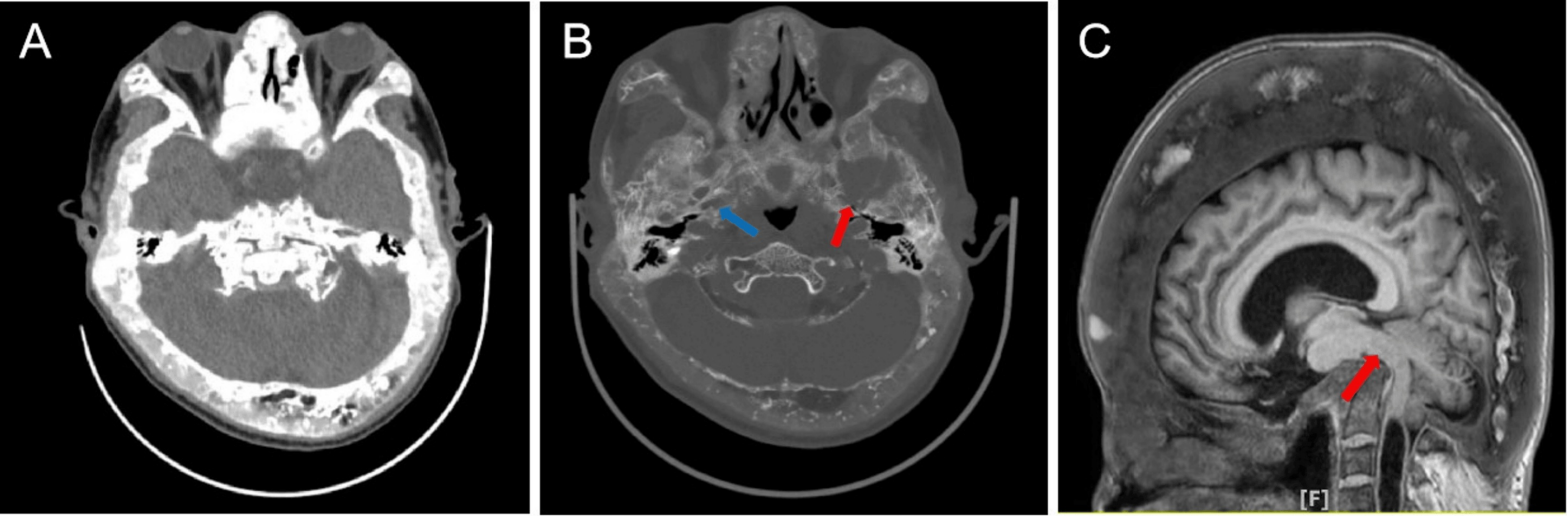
Imaging manifestations (A, B) Preoperative CT of the head. (A) Diffuse thickening of the cranial bones and uneven bone density was seen on 1.25 mm Stnd. (B) The affected foramen ovale (red arrow) appeared less distinct compared to the opposite side(blue arrow) was seen on the 1.25 mm bone. (C) MRI scans showed diffuse thickening of the cranial bones, primarily in the outer plate, with nodular and cord-like mixed signals within the diploic space. Other findings included clear gray-white matter differentiation, slightly elongated T1 and T2 signal bands around the lateral ventricles, normal ventricular and cisternal widening, reduced cerebellar volume, and brainstem distortion (red arrow). The cerebellum extends upward through the tentorial notch and downward through the foramen magnum, reaching the mid-level of the second cervical vertebra, suggesting a potential Chiari II malformation. In addition, there is evidence of syringomyelia or a cerebrospinal fluid cavity starting below C2.
